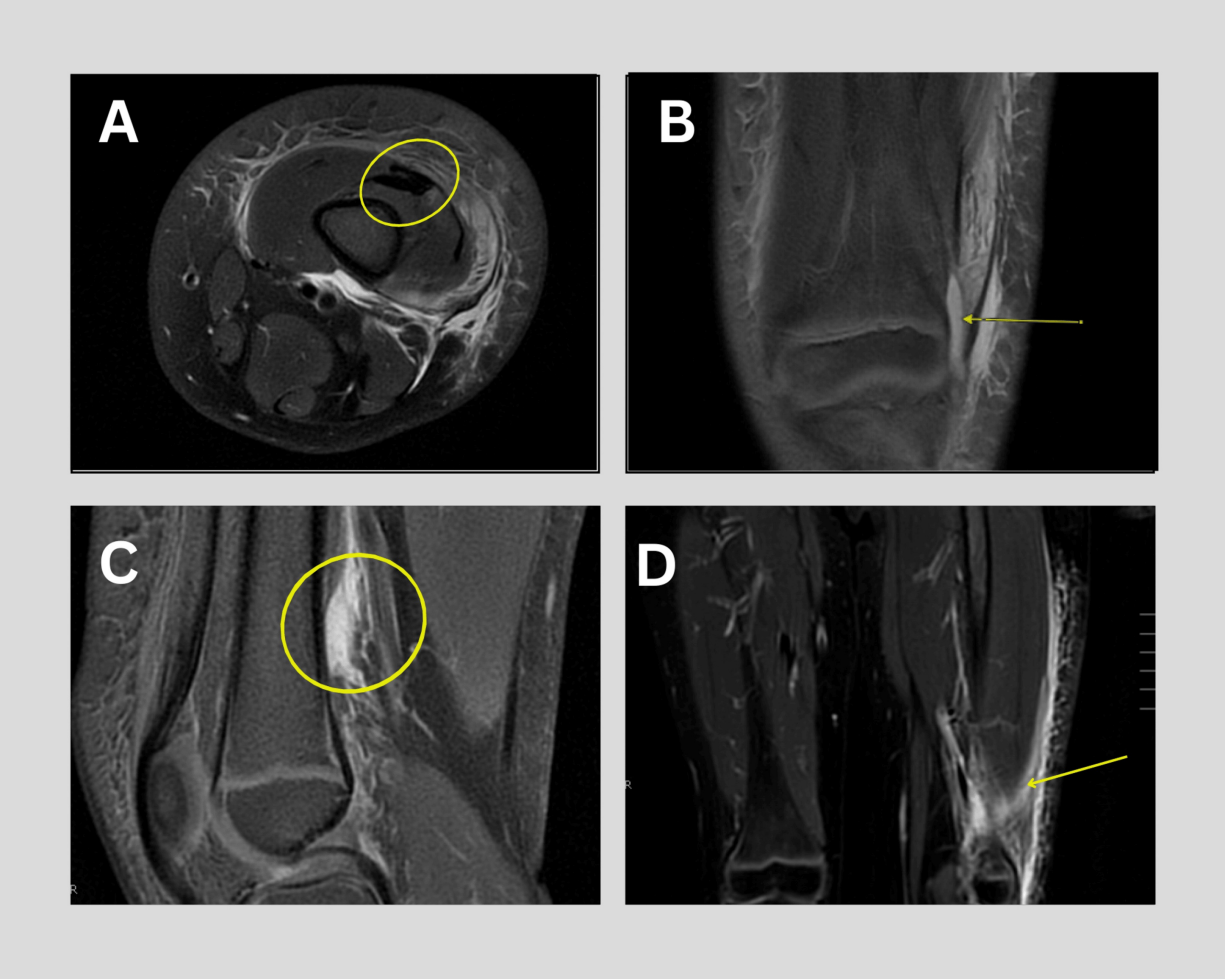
MRI of the left thigh and knee demonstrating post-vaccination inflammatory changes. (A) Axial image at the distal femur showing edema and myositis within the anterior compartment musculature; (B) Coronal image showing intramuscular fluid within the vastus lateralis with perimysial stranding; (C) Sagittal image with anterior compartment and fascial hyperintensity consistent with cellulitis and fasciitis; (D) Coronal image revealing soft tissue inflammation extending into the lateral posterior compartment.
